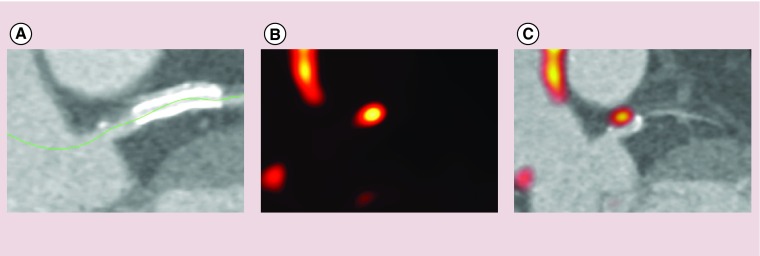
^18^F-sodium fluoride and plaque rupture. Patient presenting with acute myocardial infarction. (A) Computed tomography demonstrating high-risk features (spotty calcification and low attenuation plaque) in that region. (B & C) The culprit plaque also demonstrated increased ^18^F-fluoride positron emission tomography activity on hybrid positron emission tomography/CT image.
